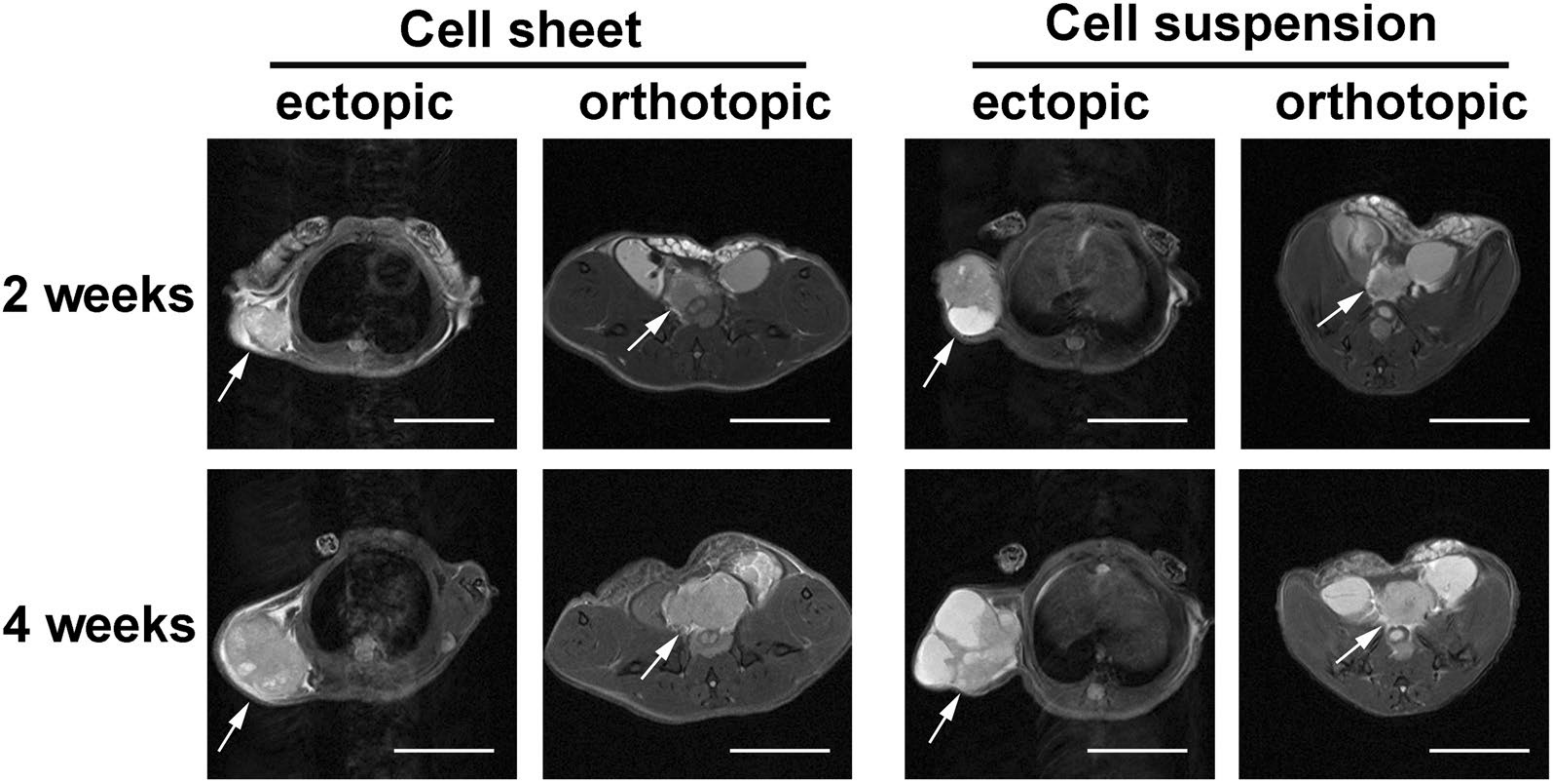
MRI in vivo. MRI was performed at 2 and 4 weeks after transplantation. White arrows represent the area of the tumor. Scale bar: 1 cm
